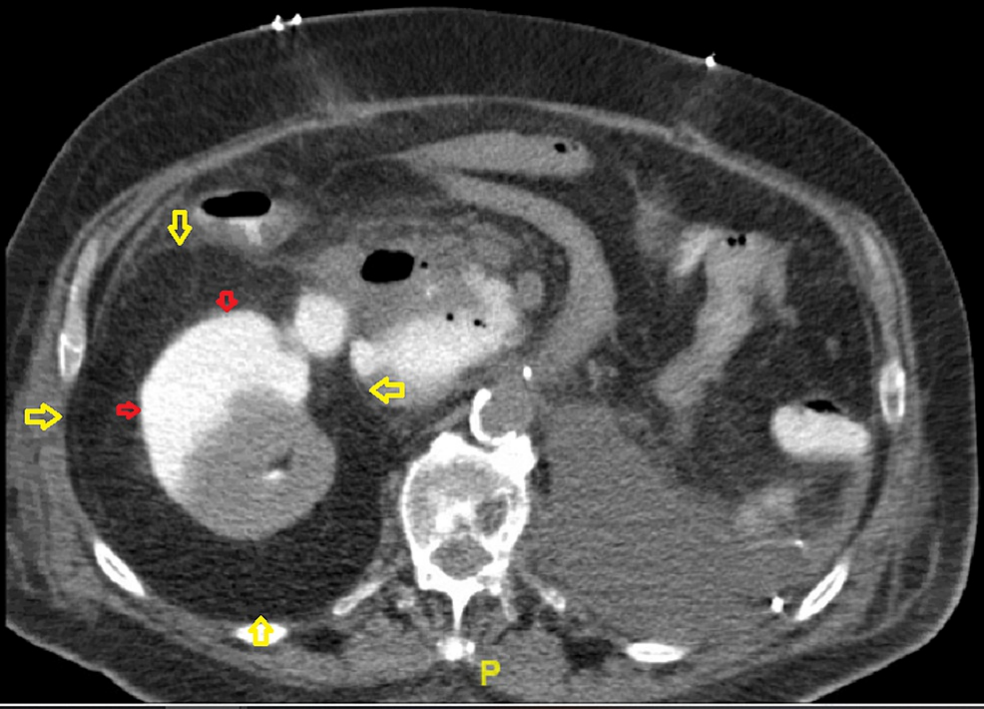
CT abdomen showing large perirenal fluid collection (yellow arrows) as well as subcapsular fluid collection (red arrows)
